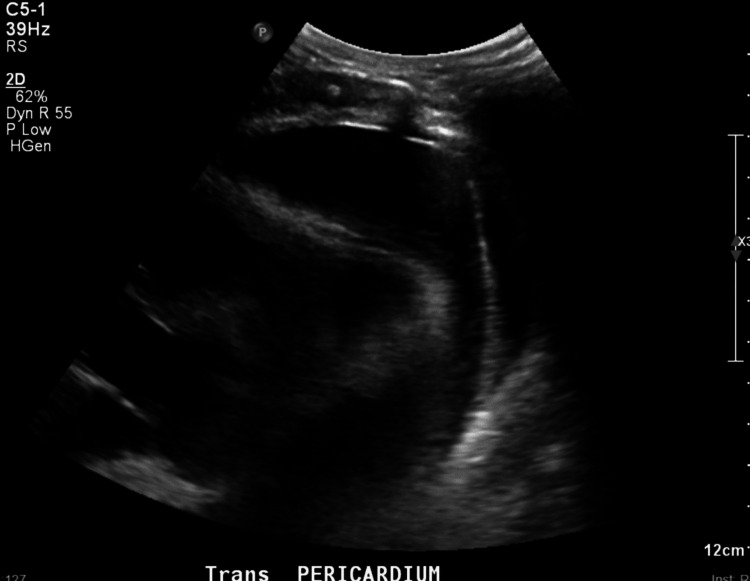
Transthoracic echocardiogram The image shows malignant pericardial effusion space prior to pericardiocentesis with the removal of 120 mL of fluid
